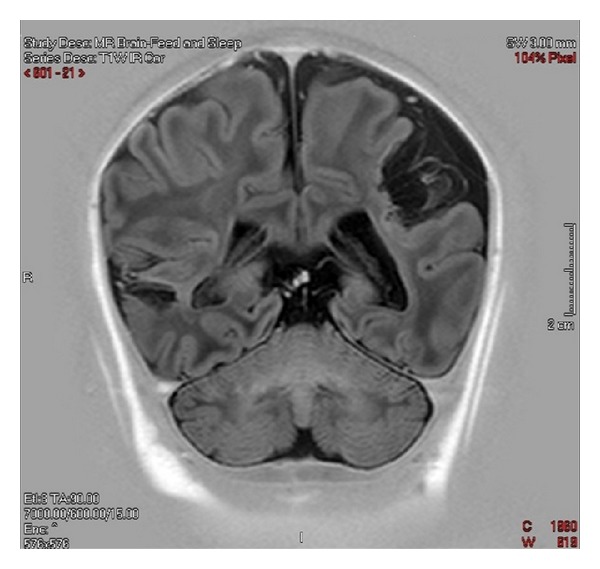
T1-weighted imaging four weeks postpartum showing significant lesions at the left parieto-occipital area.
